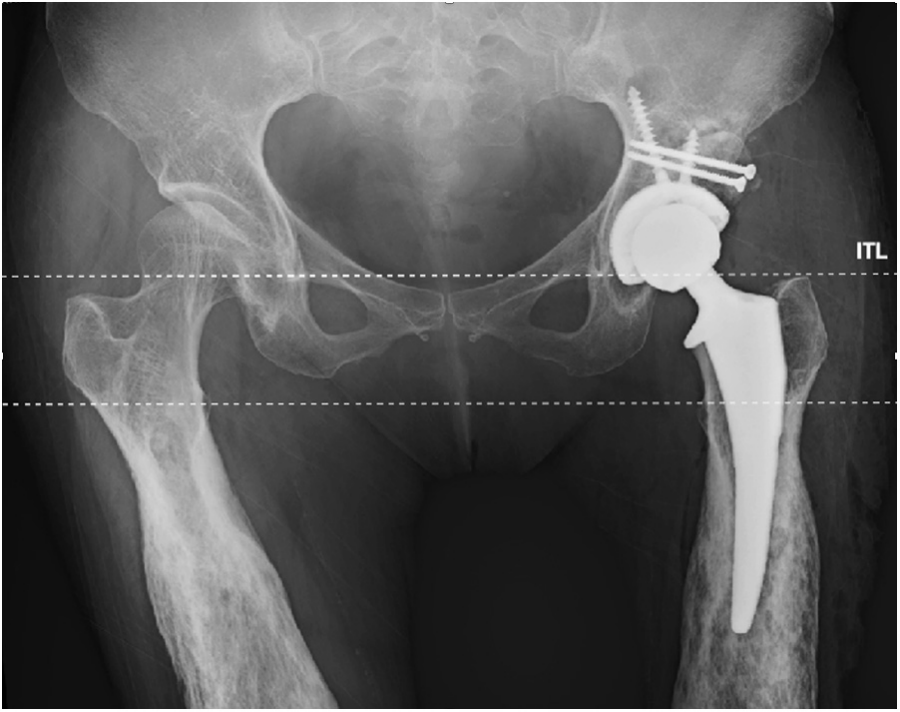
Postoperative anteroposterior radiograph demonstrating restoration of leg lengths and offset. ITL, interteardrop line.
